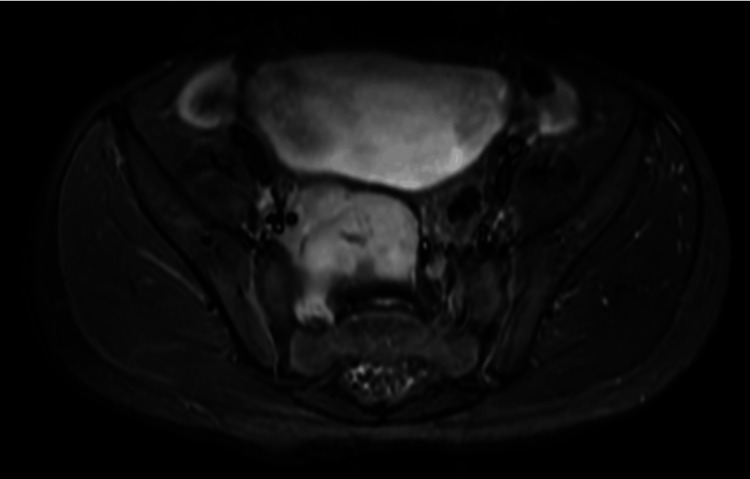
Axial image of MRI showed a solid mass on the right side of the pelvis posterior to the urinary bladder and seen extending to the right sacral foramina.
